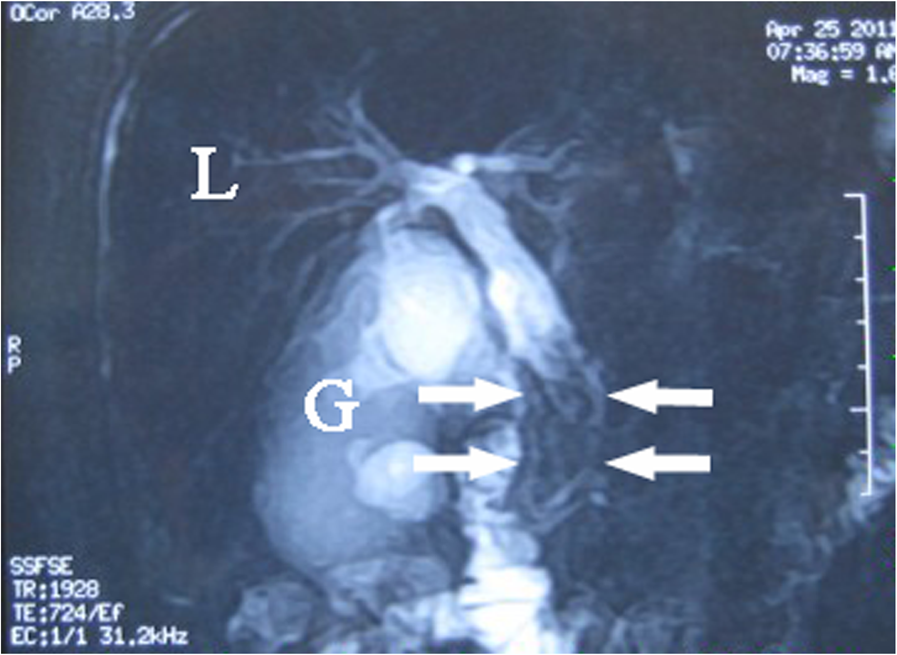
Magnetic resonance imaging findings on 25 April 2011. Magnetic resonance cholangiopancreatography, showing irregular hypointense areas in the common bile duct (white arrows) with obvious dilation of the distal bile ducts. G, gallbladder; L, liver.
